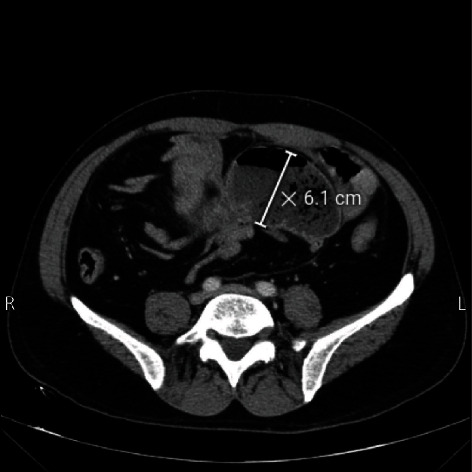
Axial image of contrast-enhanced CT shows dilated small bowel loop, a sign of small bowel obstruction.
